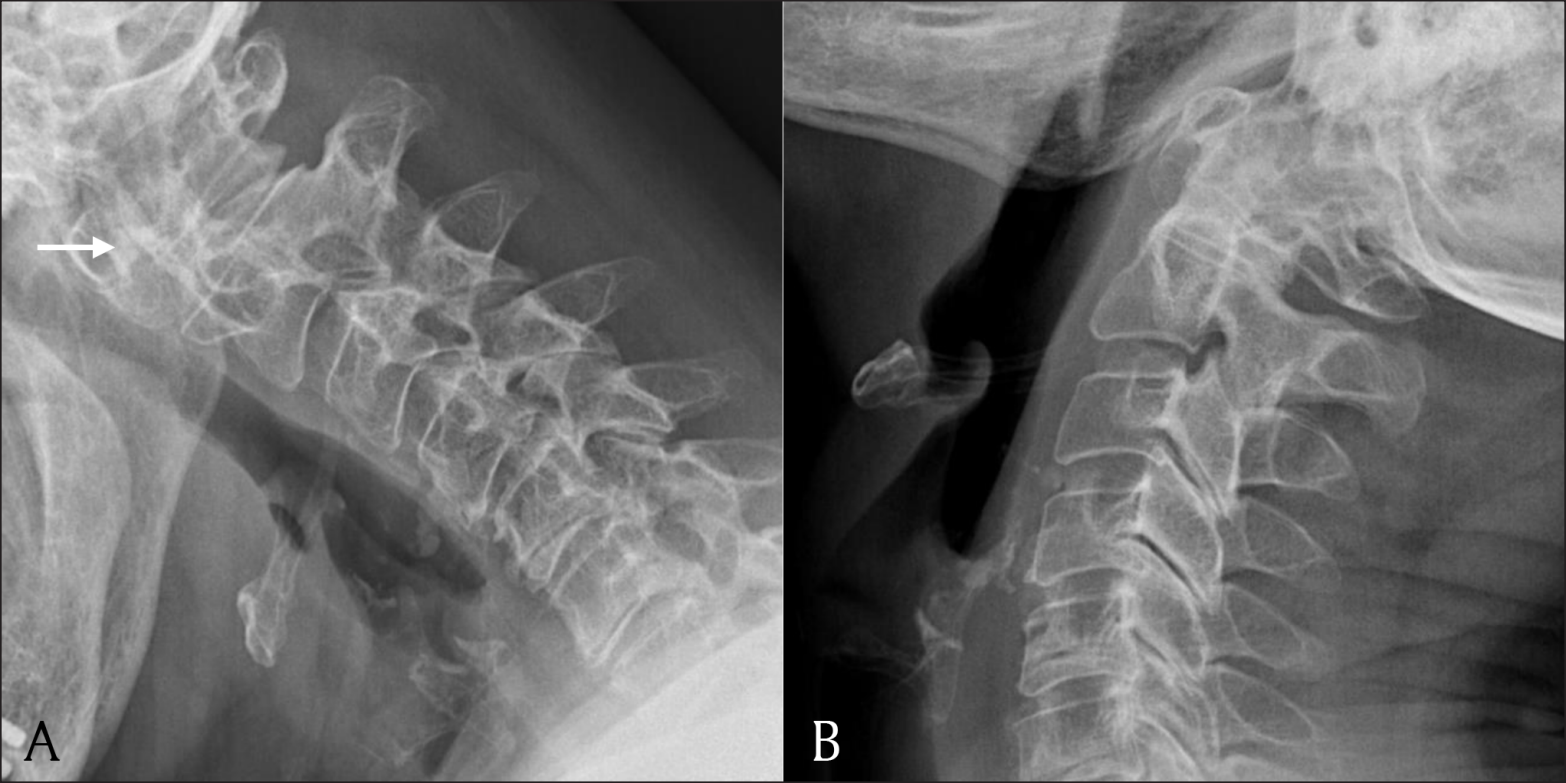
A = lateral view of the cervical spine in flexion; B = lateral view of the cervical spine in extension. Plain radiograph showing a lateral view of the cervical spine in flexion and extension. Note widening of the atlantodental interval in flexion (white arrow).
